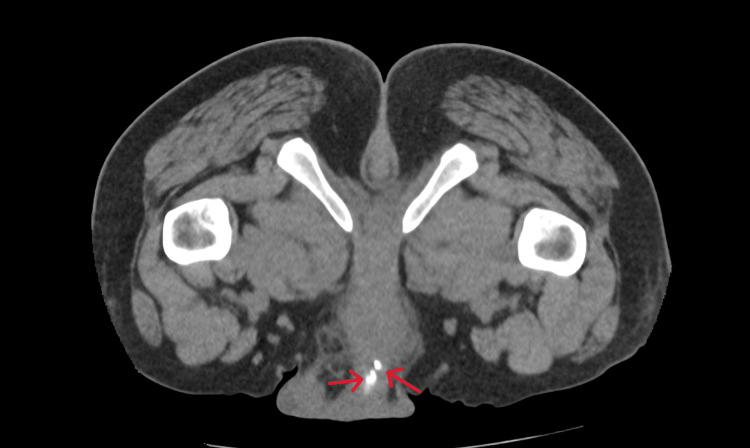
Computed tomography of the kidneys, ureters and bladder showing multifocal calcifications in the urethra suggestive of stones (red arrows).
